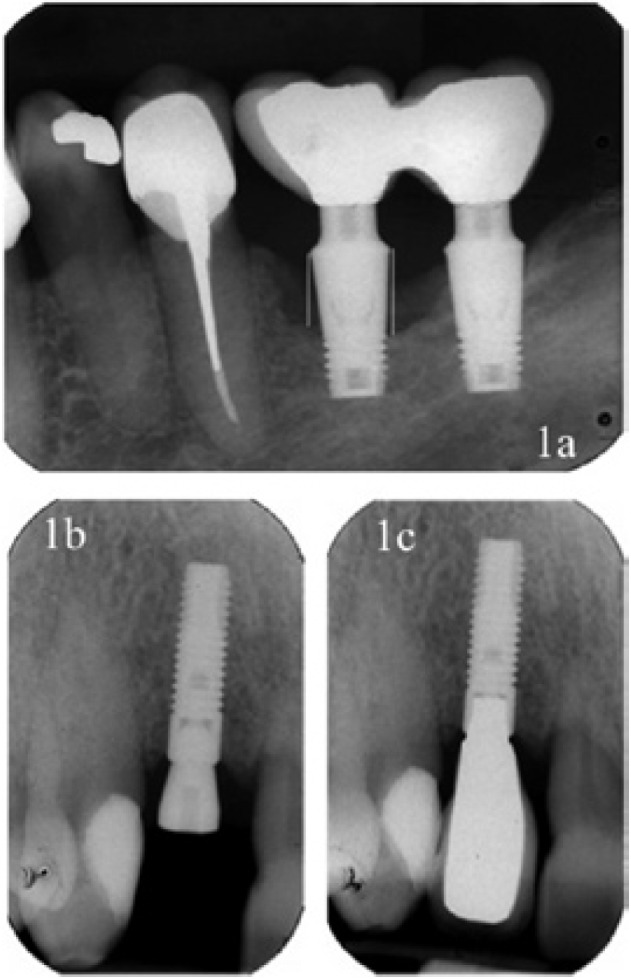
a) X-ray containing the references used in the measurements made from the implant shoulder to bone level, both mesially and distally. b) X-ray at the time of implant loading, showing that the bone margin coincides with the implant shoulder. c) X-ray after one year of functional loading in a patient who has received supportive periodontal therapy. The maintenance of the bone level can be observed.
